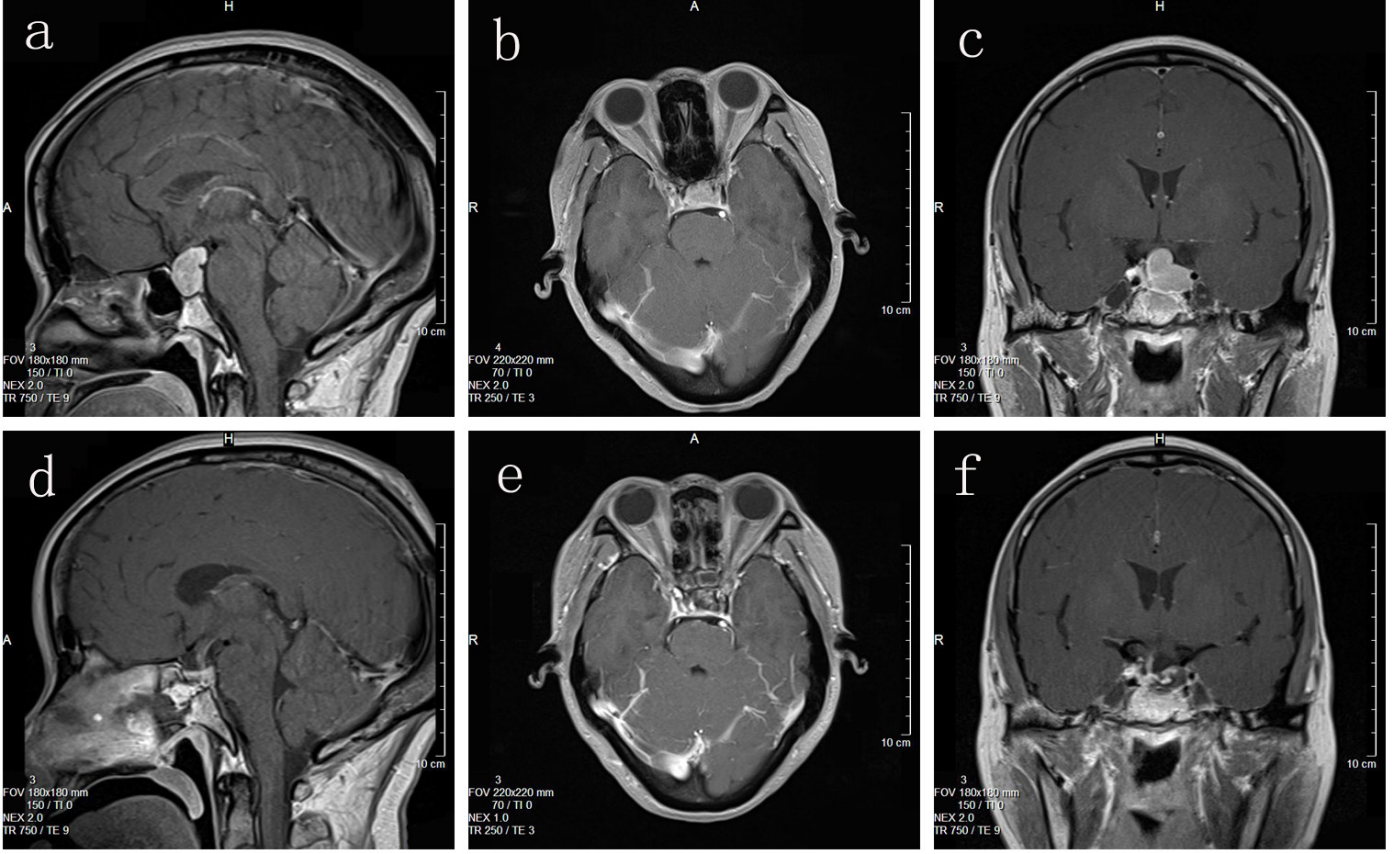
(a, b, c) Preoperative MRI imaging; (d, e, f) Postoperative MRI imaging
